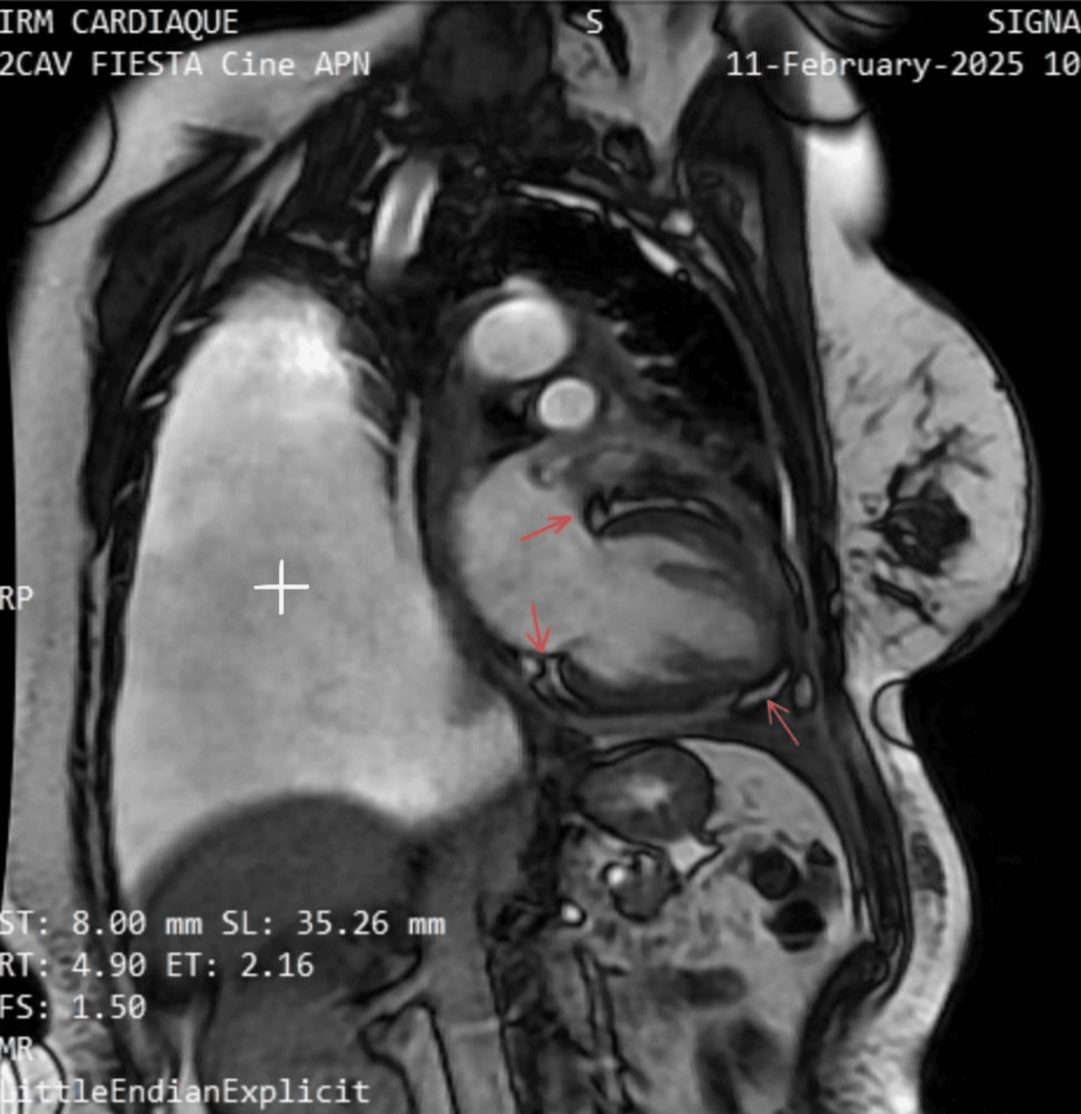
Cardiac MRI in a cine two-chamber view shows subacute inflammatory pericarditis (red arrows) with an evolving constrictive pattern affecting ventricular filling and a large pleural effusion (white plus) MRI: magnetic resonance imaging
